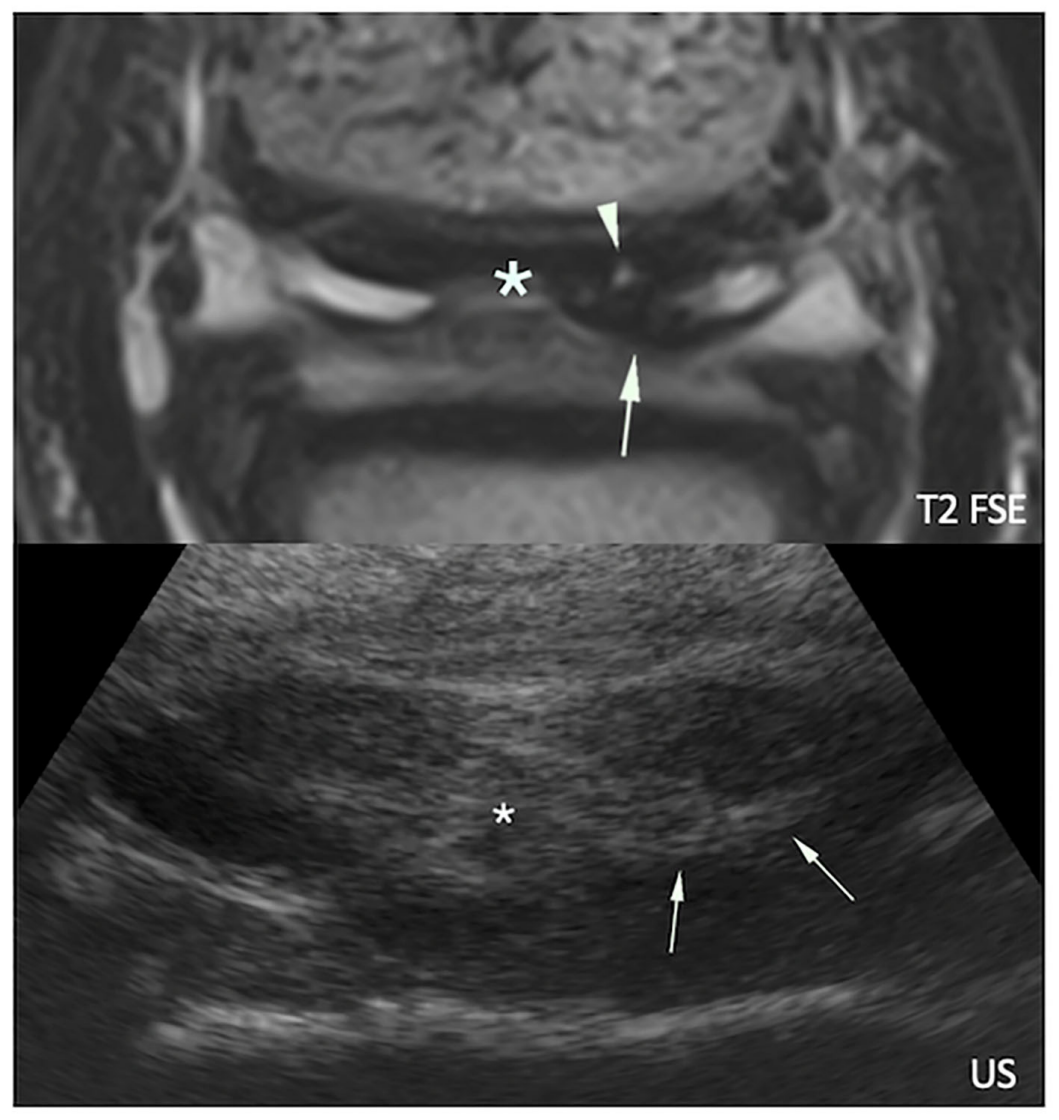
Transverse magnetic resonance (MR, top) and ultrasonographic (bottom) images of a right front foot focused on the deep digital flexor tendon (DDFT) and navicular bursa: lateral is to the right and dorsal to the bottom. The navicular bursa is distended by a moderate amount of fluid, and there is marked thickening of the mesotendon (asterisks). Proliferated soft tissue material (arrows) is visible adjacent to a MR hyperintense dorsal lesion of the DDFT lateral lobe (arrowhead).
